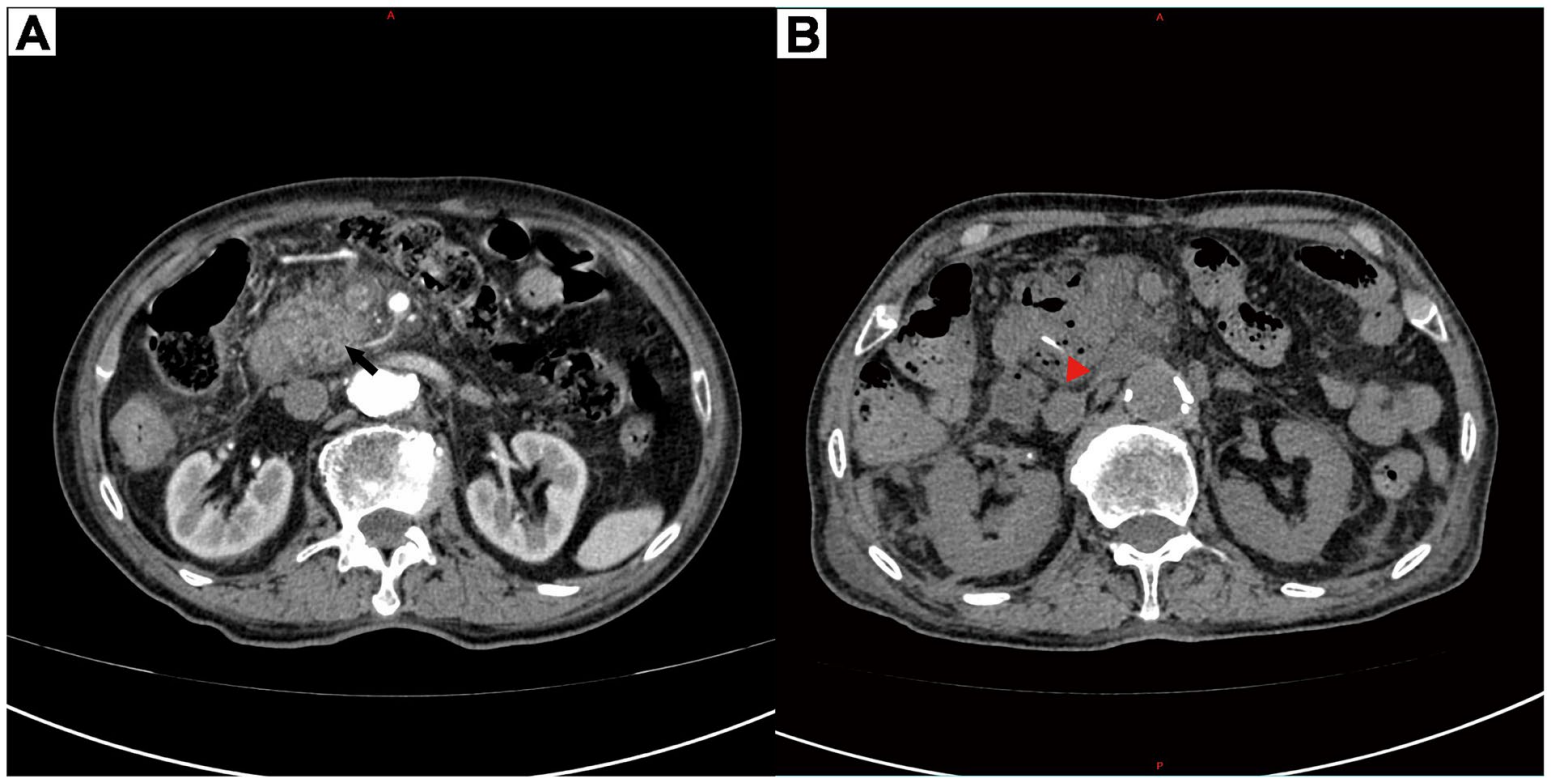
(A) Contrast-enhanced computed tomography showed fullness of the head of the pancreas, peripheral oozing, and blurring of the surrounding fat space (black arrow). (B) Contrast-enhanced computed tomography showed a localized hyperdense shadow (red triangle) in the head-duodenal region of the pancreas with a long diameter of approximately 21 mm and a fixed morphology.
